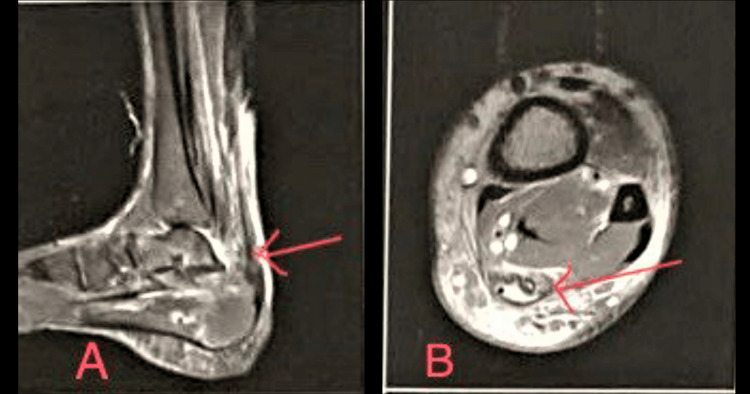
T2-weighted MRI showing hyperintense signal suggestive of Achilles tendon rupture. A: sagittal section; B: axial section.
